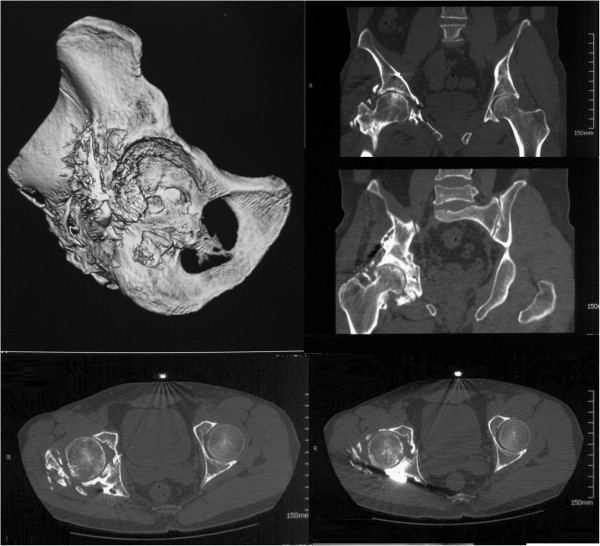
CT scans and reconstruction at the final follow-up show more than 50% of joint space existed and the femoral head was mostly congruent with the acetabulum. Although subchondral cysts and minimal signs of arthritis can be observed in the femoral head and acetabulum, no evidence of collapse or necrosis was found either in the femoral or acetabular subchondral bone.
